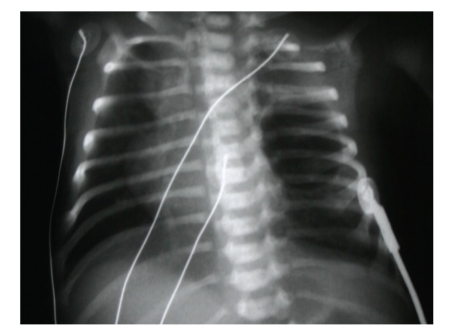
Roentgen imaging of the lungs after birth.
